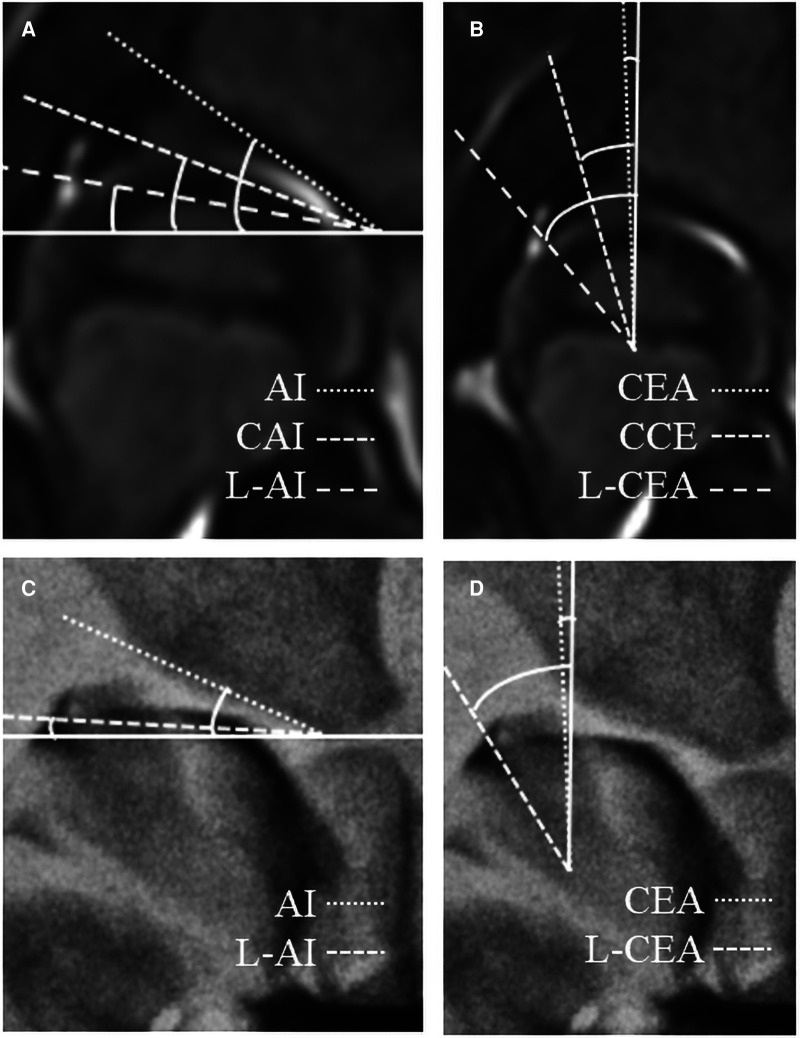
Some cartilaginous indicators measured on the coronal plane of MRI (A,C) and the anteroposterior film of hip arthrography (B,D). AI, acetabular index; CEA, centre-edge angle; CAI, cartilaginous acetabular index; CCE, cartilaginous center-edge angle; L, labral.
